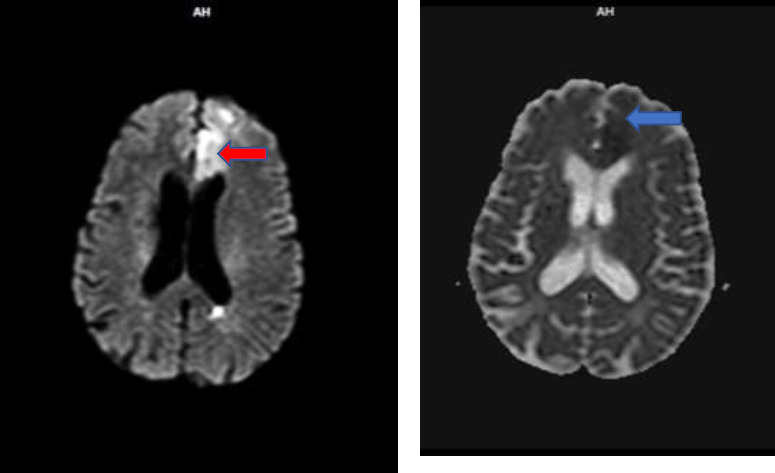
Magnetic resonance imaging (MRI) showing 4.5 x 1.8 cm wedged-shaped cortical -a subcortical region in the left frontal lobe, which is hyperintense (red arrow) on diffusion-weighted imaging (DWI) and shows subtle hypointensity (blue arrow) on apparent diffusion coefficient (ADC) sequence
